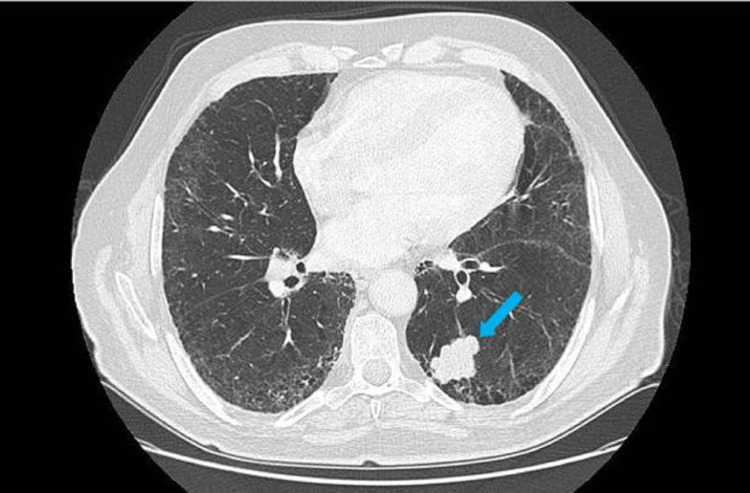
A mass (blue arrow) seen in the left posterior-basilar lung on chest CT .
